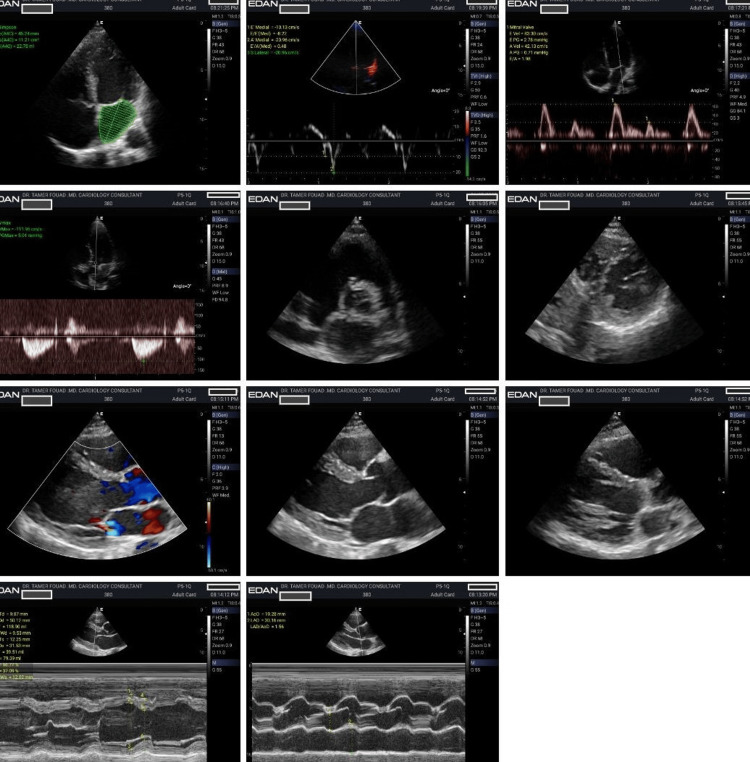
Baseline echocardiogram prior to SGLT2 inhibitor initiation SGLT2: sodium-glucose cotransporter 2 This figure represents a case with a baseline echocardiography demonstrating left atrial enlargement and diastolic dysfunction prior to treatment. The study is notable for a significantly dilated left atrium. The mitral inflow pattern and tissue Doppler indices show restrictive physiology, indicative of advanced diastolic dysfunction and high left atrial pressure
